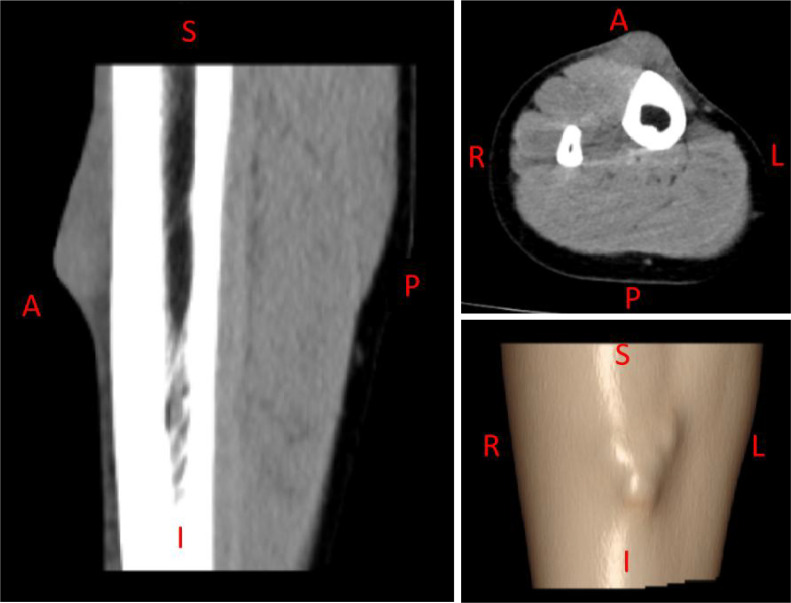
Preoperative CT images. A patchy, homogeneous, hyperdense lesion (approx. 34 mm × 11 mm × 45 mm; CT value ~38 HU) with ill-defined margins is seen anterior to the right tibia. A, anterior; P, posterior; S, superior; I, inferior; R, right; L, left.
